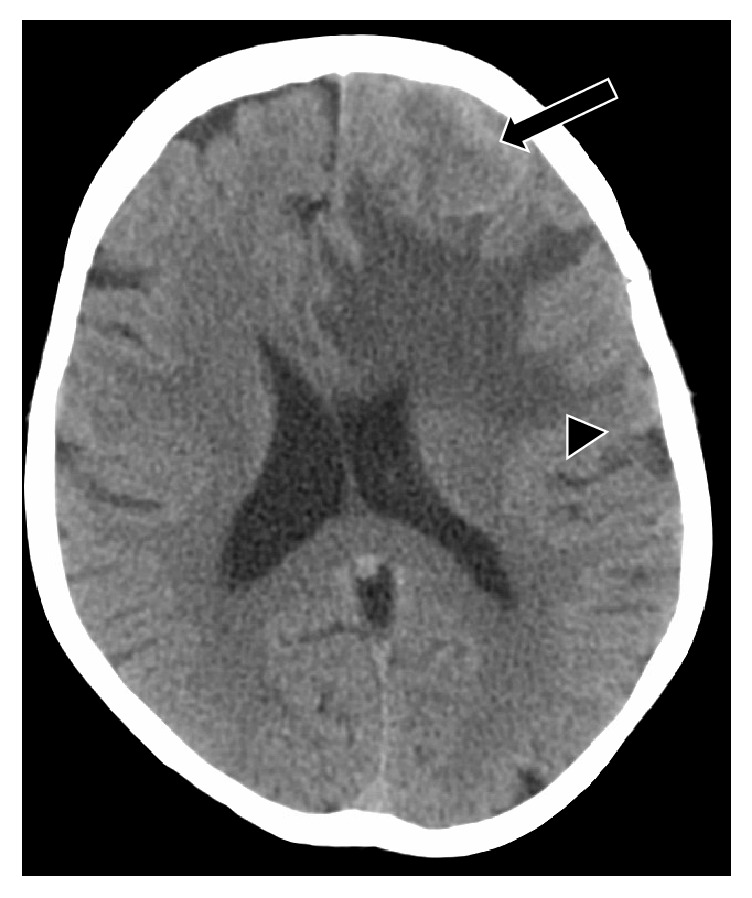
At initial presentation with altered mental status, CT head without contrast showed a left frontal mass (arrow) with surrounding edema (arrow head) and midline shift.
